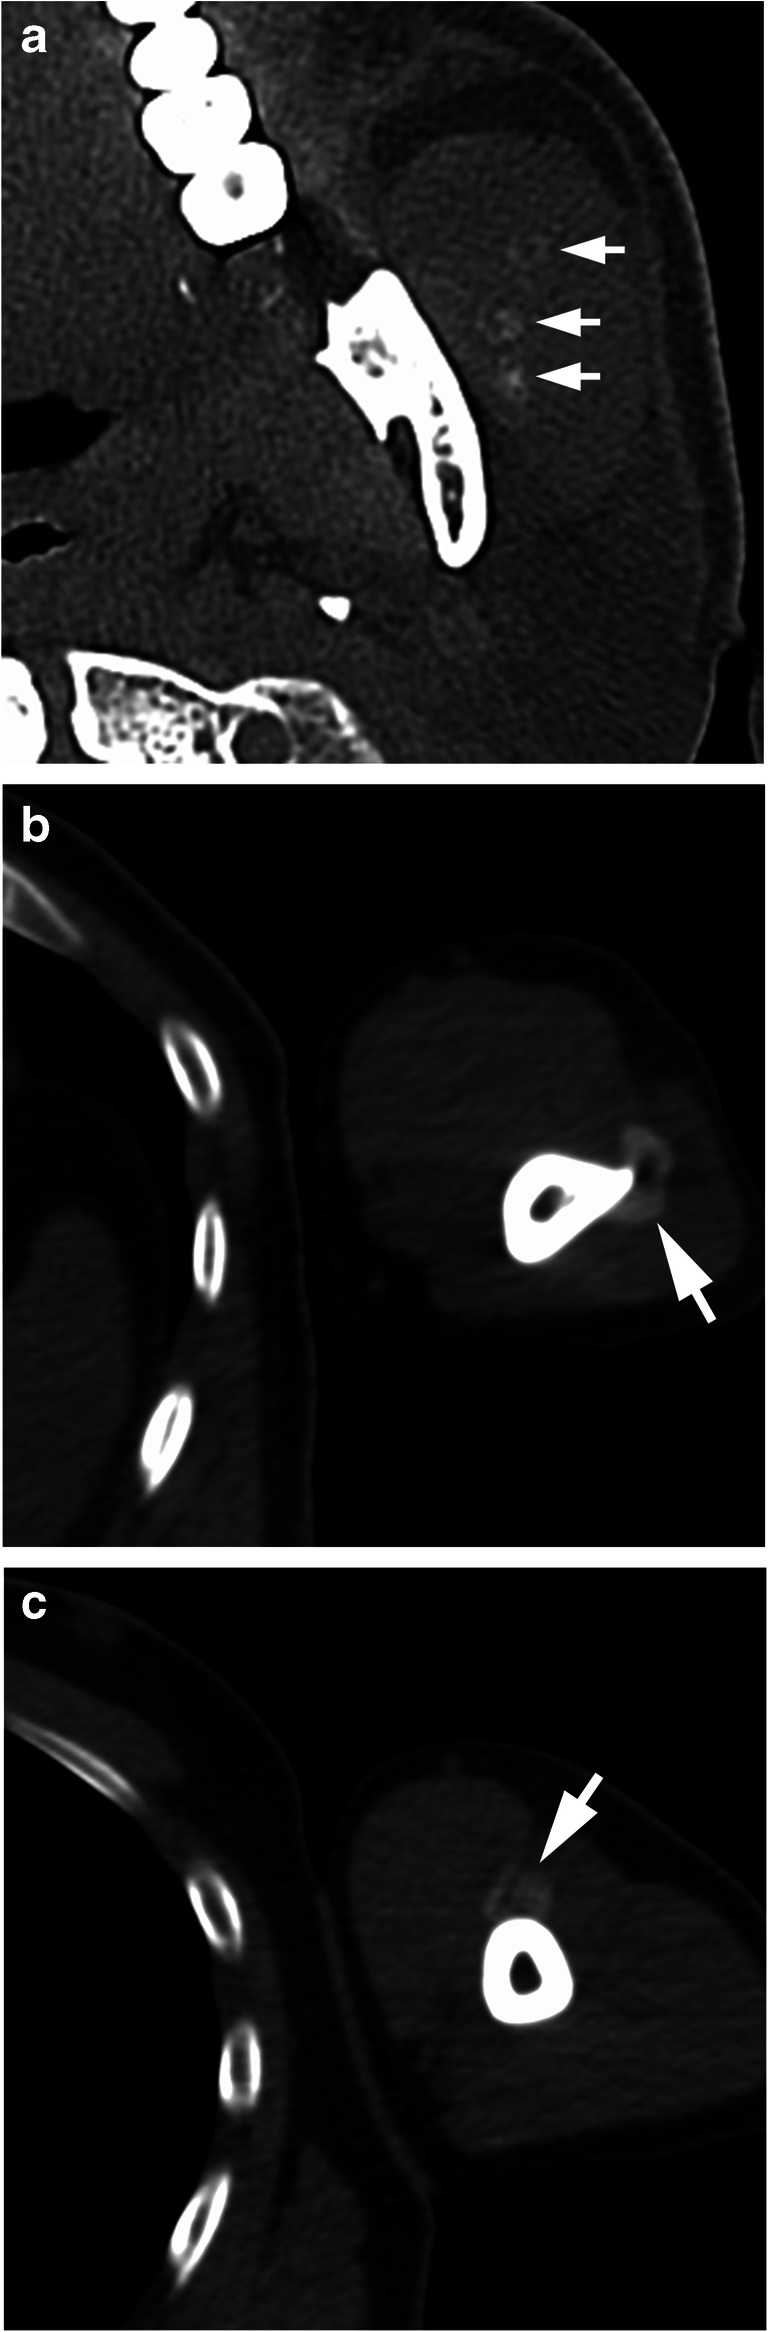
Conventional axial computed tomography images with bone-window setting show faint but circled calcifications in the a left masseter (arrows), b triceps brachii (arrow), and c biceps brachii (arrow) muscles
